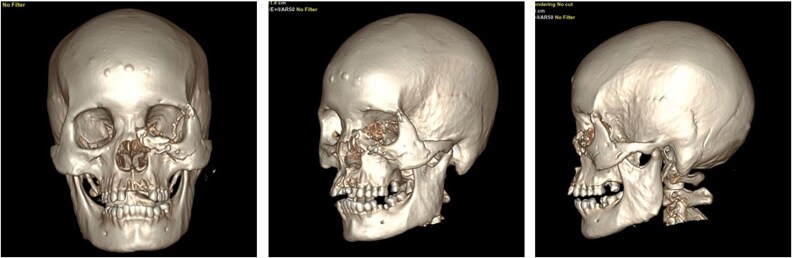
Postoperative CT evaluation demonstrating successful bony consolidation and stable wire fixation.
